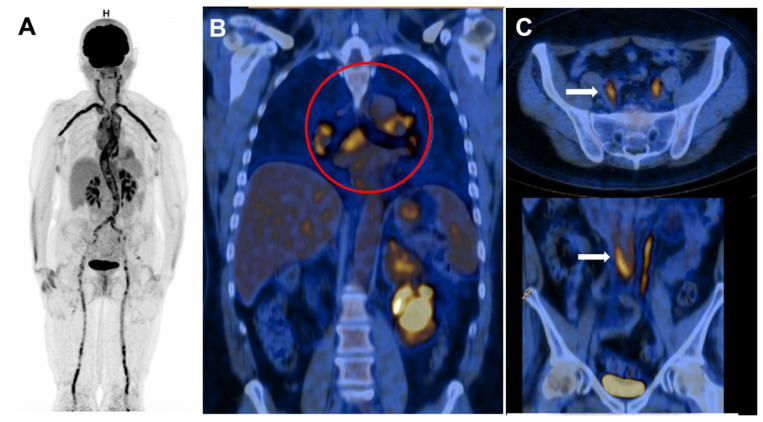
^18^FDG PET in giant cell arteritis (GCA) (A) and IgG4 related-disease (IgG4-RD) vasculitis (B,C). Panel A shows intense large vessel vasculitis of the thoracic and abdominal aorta, carotid arteries, subclavian arteries, iliac and femoral arteries in a patient with active GCA. Panel (B) shows mediastinal adenopathy (red circle) in IgG4-RD. Panel (C) shows tracer uptake in abdominal aorta and iliac arteries (white arrows) in IgG4-RD.
